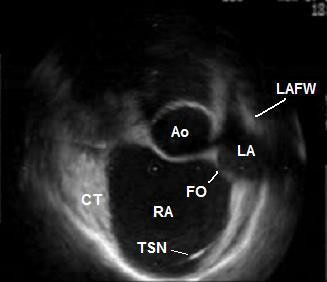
ICE during atrial diastole before the jump of the transseptal needle into the oval fossa. In this patient the LA cavity has a crescent-like shape at this time point of the cardiac cycle. Ao – non-coronary sinus of the aorta; CT – terminal crest; FO – oval fossa; LA – left atrium; LAFW – left atrial free wall; RA – right atrium; TSN – transseptal needle.
